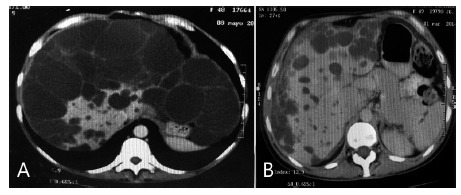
A) Pre-operative CT-Scan shows grade III APLD; B) control CT-Scan shows important hypertrophy of remanent liver parenchyma and several small size cysts remaining
